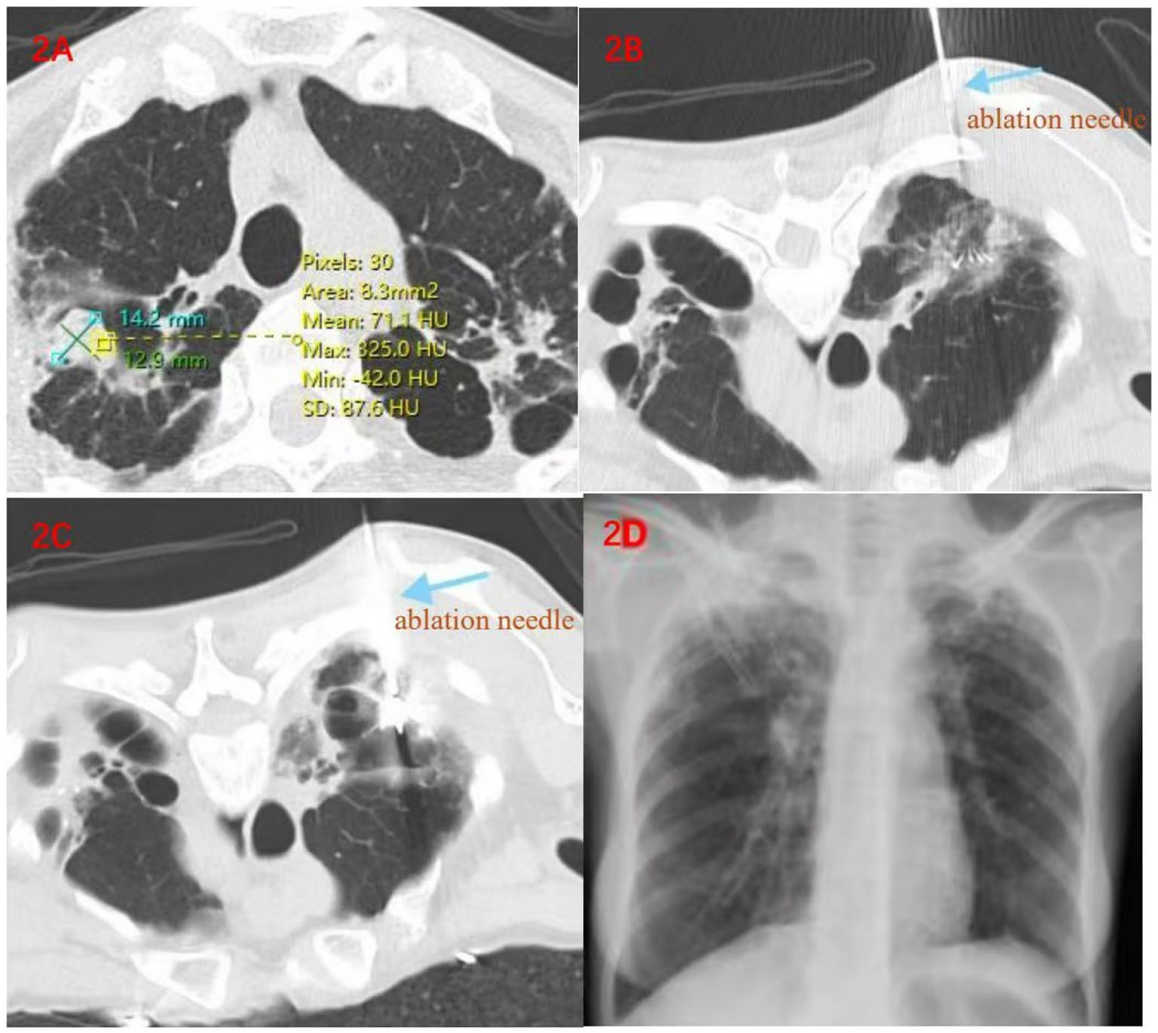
(A) On February 17, 2023, before the operation, a cavity was formed in the upper lobe of the right lung, and nodular soft tissue shadows and calcification foci could be seen inside it; (B,C). The process of radiofrequency ablation; (D) A follow-up X-ray examination on the second day after the operation showed no changes such as pneumothorax or exudation, indicating that the ablation was carried out smoothly.
